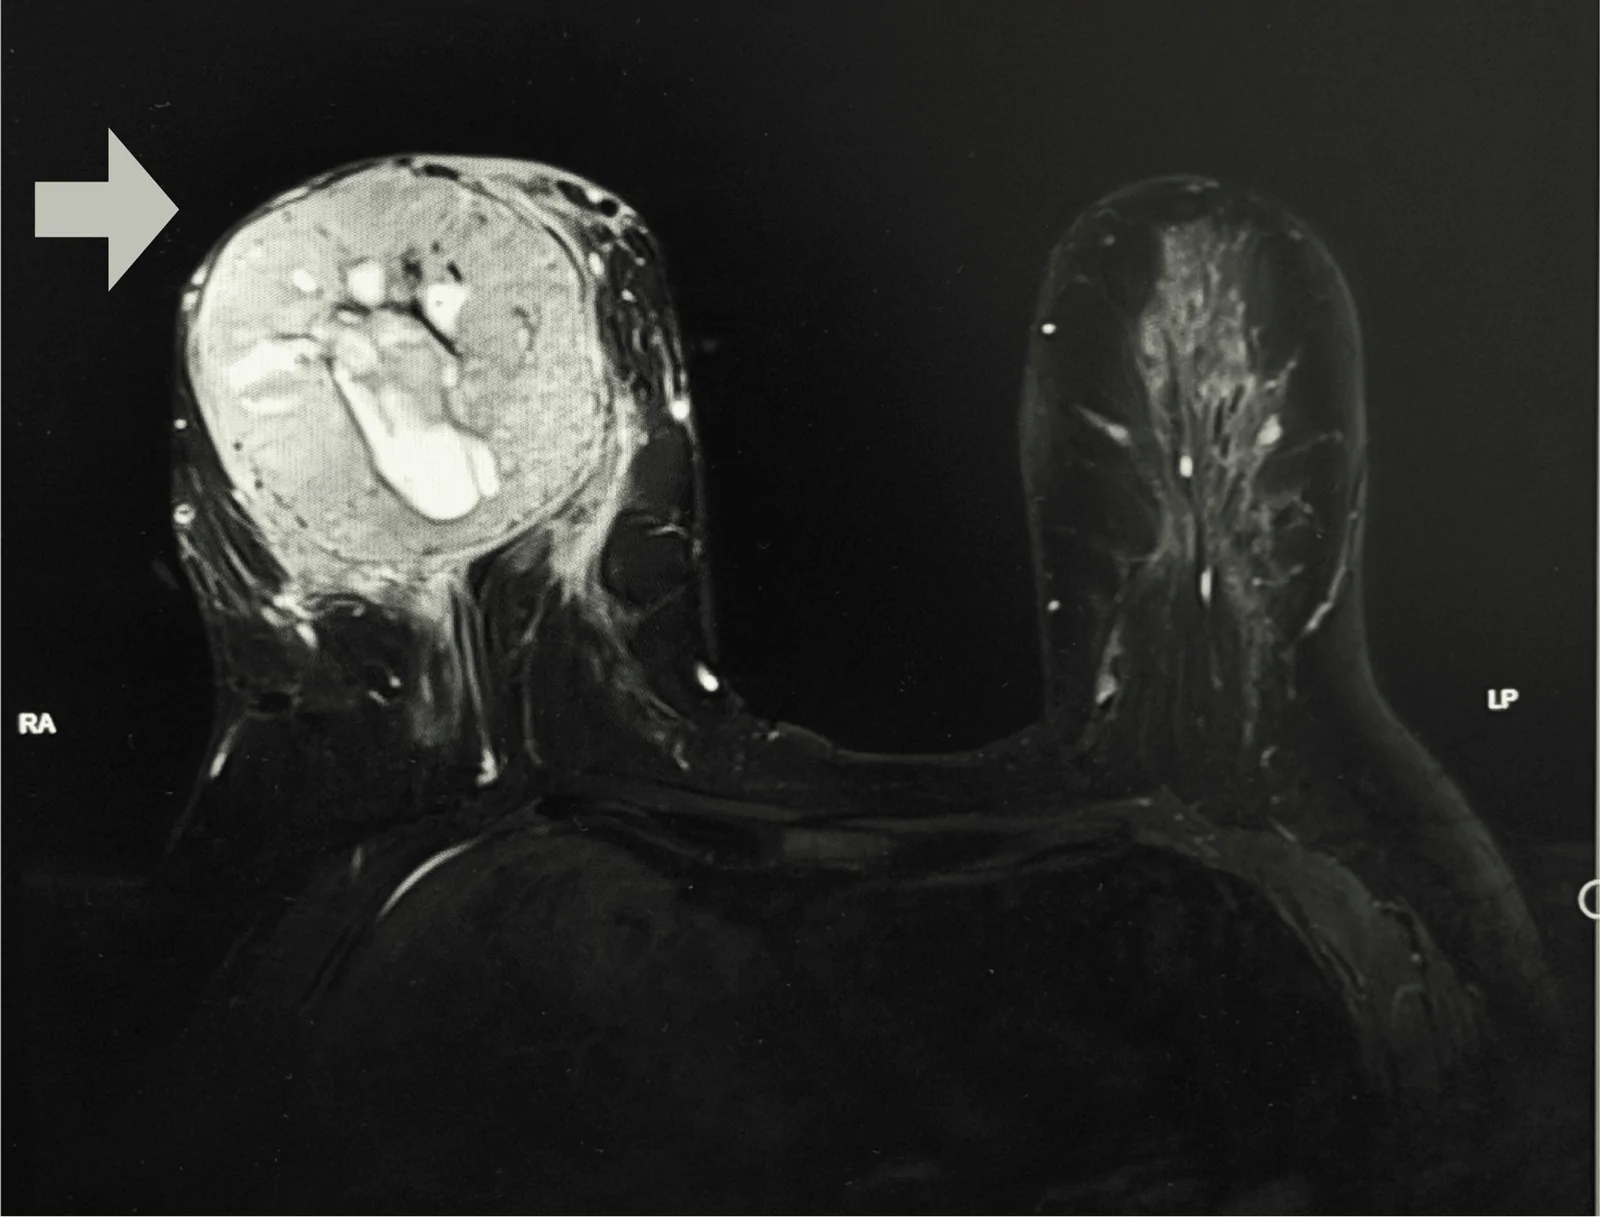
MRI: Index mass measuring 8.2 x 8 x 7.6 cm in the right breast (arrow). The mass was seen extending to all breast quadrants, inseparable from overlying skin at some points and in close proximity to the nipple (with retraction). MRI: magnetic resonance imaging.
